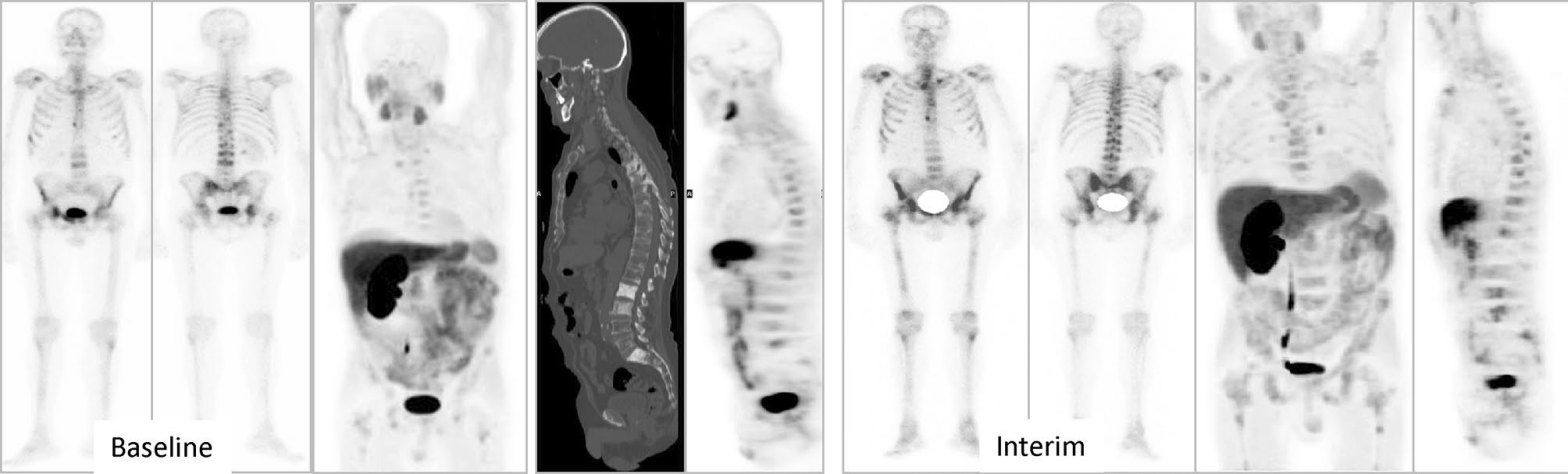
Patient ≠ 4. Baseline BS and FCH PET/CT shows polimetastatic disease with progression on interim images. 66 years old man, Gleason 9, ^223^Ra in second line, baseline PSA: 57 ng/ml. PFS and OS of 3 and 14 months, respectively.
